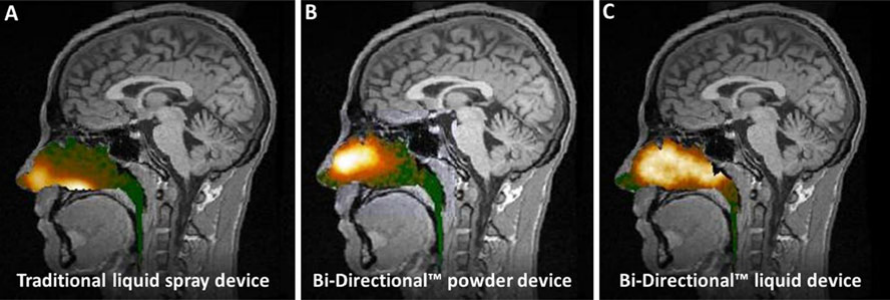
Gamma camera image information (logarithmic “hot iron” intensity scale) from the nasal cavity is superimposed on the corresponding sagittal MRI section. The images are from the same subject and present deposition 2 min after delivery using (a) a traditional liquid spray, (b) the breath-powered Bi-Directional™ powder device, and (c) the breath-powered Bi-Directional™ liquid spray device incorporating the same spray pump as used in a. The initial deposition following traditional spray was greatest in the lower anterior regions of the nose, whereas deposition with the Bi-Directional™ delivery devices was greatest in the upper posterior regions of the nose. The less broad distribution in b following breath-powered Bi-Directional™ powder device is believed to be due to the slower clearance for powder the first 6–8 min, reflecting the dissolution of the powder into the mucosal layer. a and b have been published previously, and they are reprinted with permission from the publisher [14]
